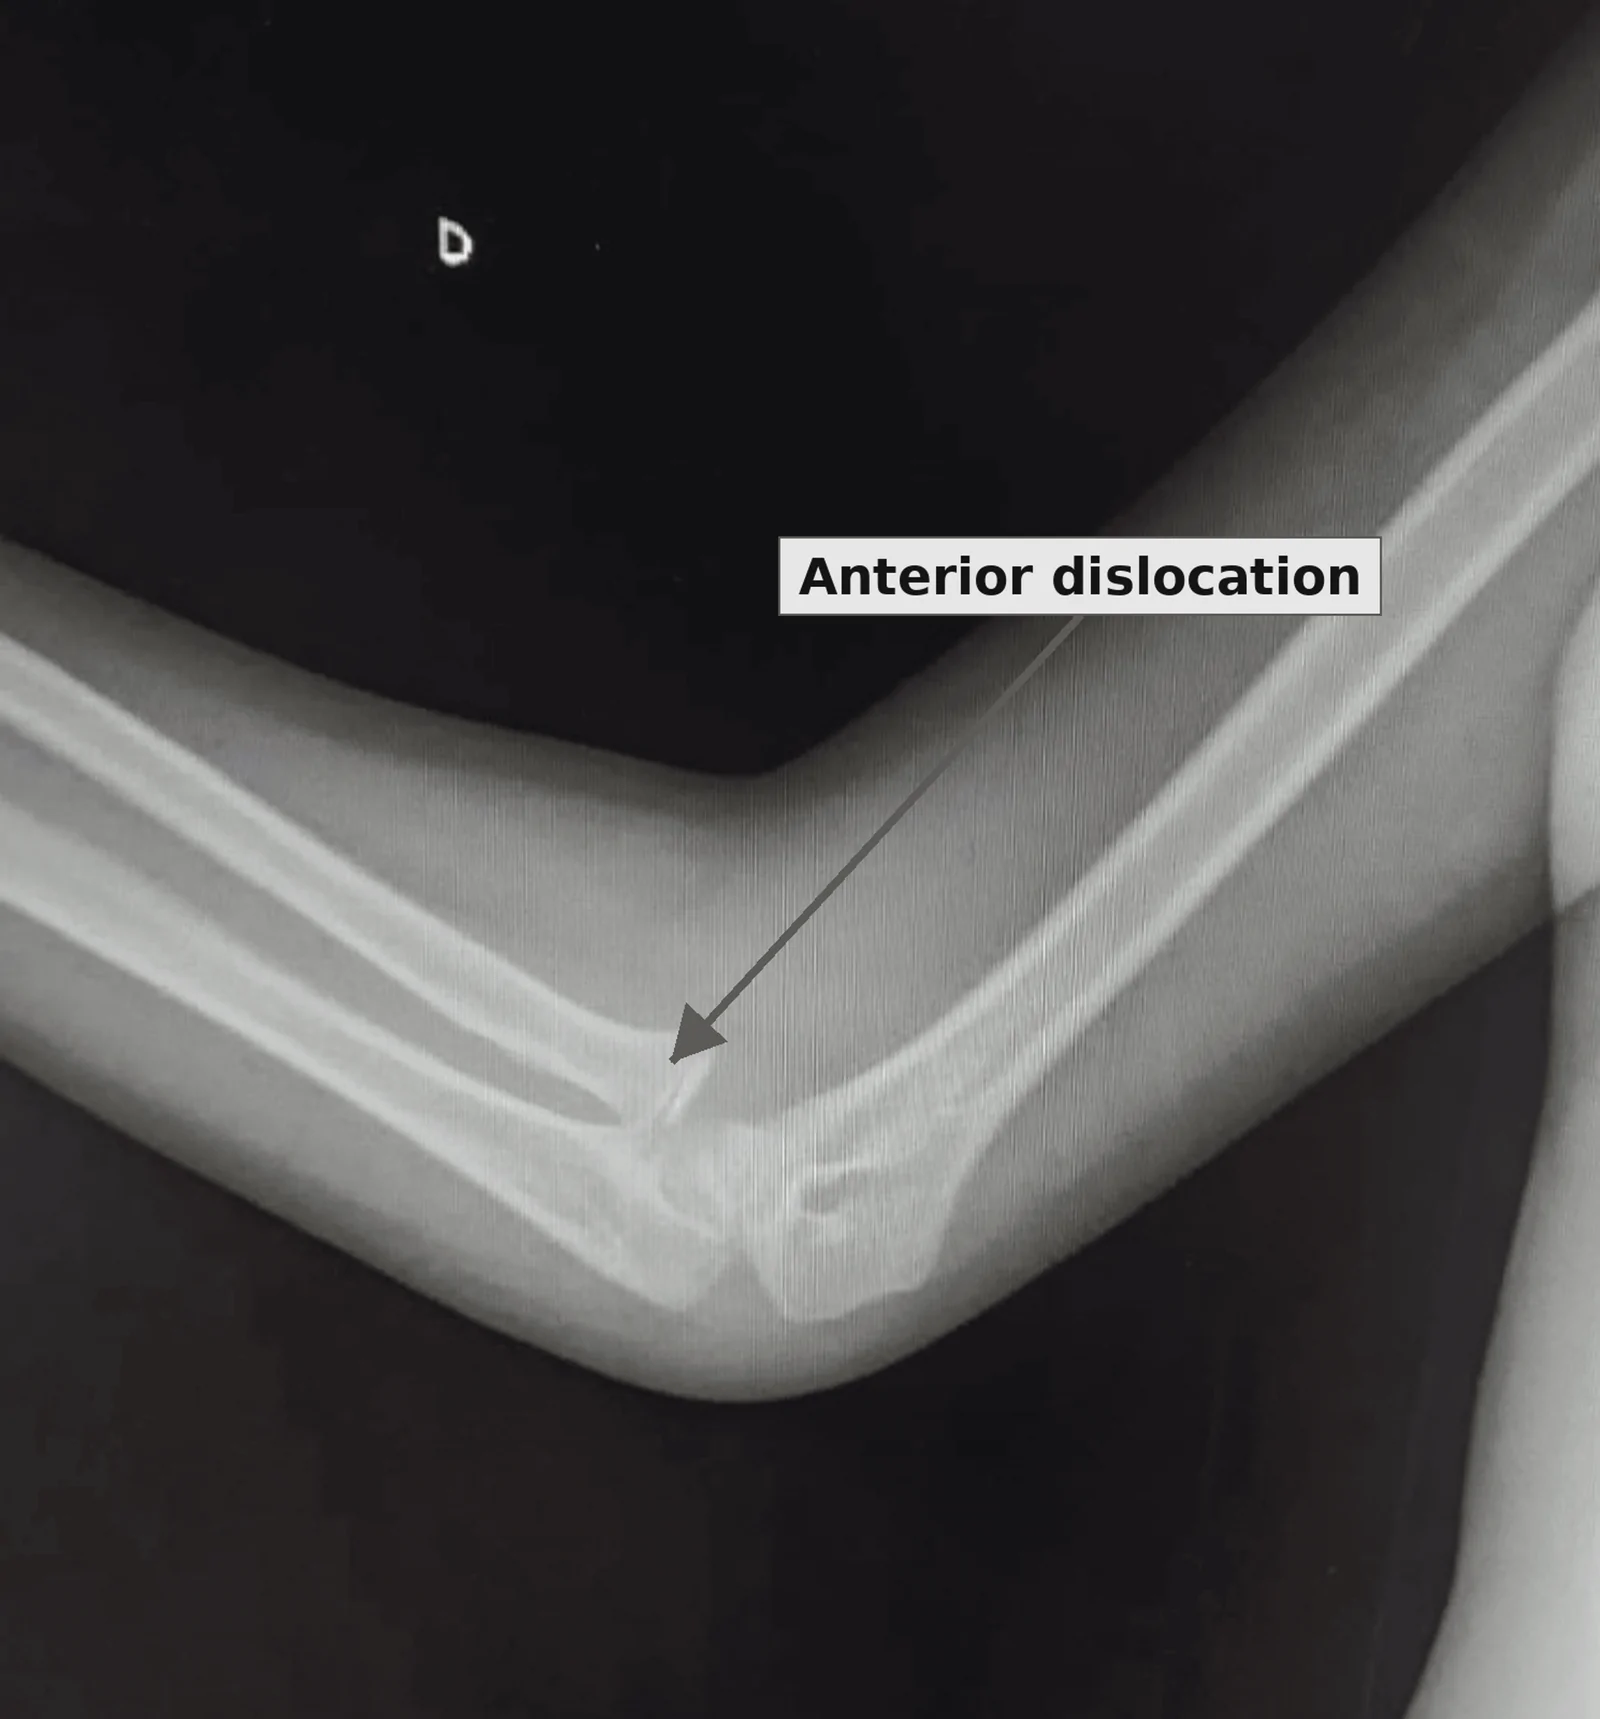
Lateral radiograph of the right elbow demonstrating anterior dislocation of the radius and ulna prior to reduction.
